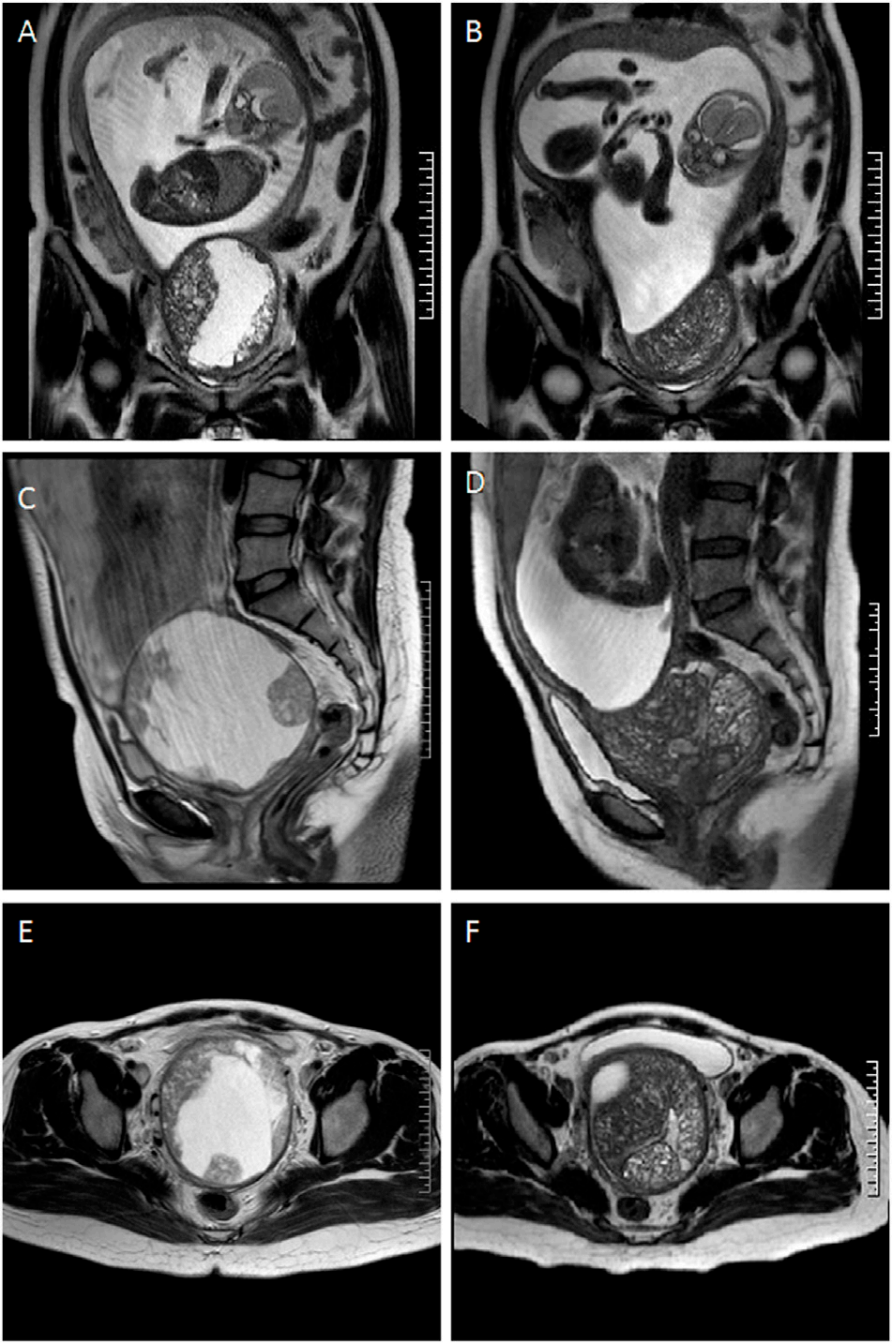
Comparison of the magnetic resonance images of the tumor at the coronal, sagittal, and axial plane before and after rupture. (A), (C) and (E) tumor at 20 weeks (before rupture) was shown. (B), (D) and (F) tumor at 22 weeks (after rupture) was shown.
